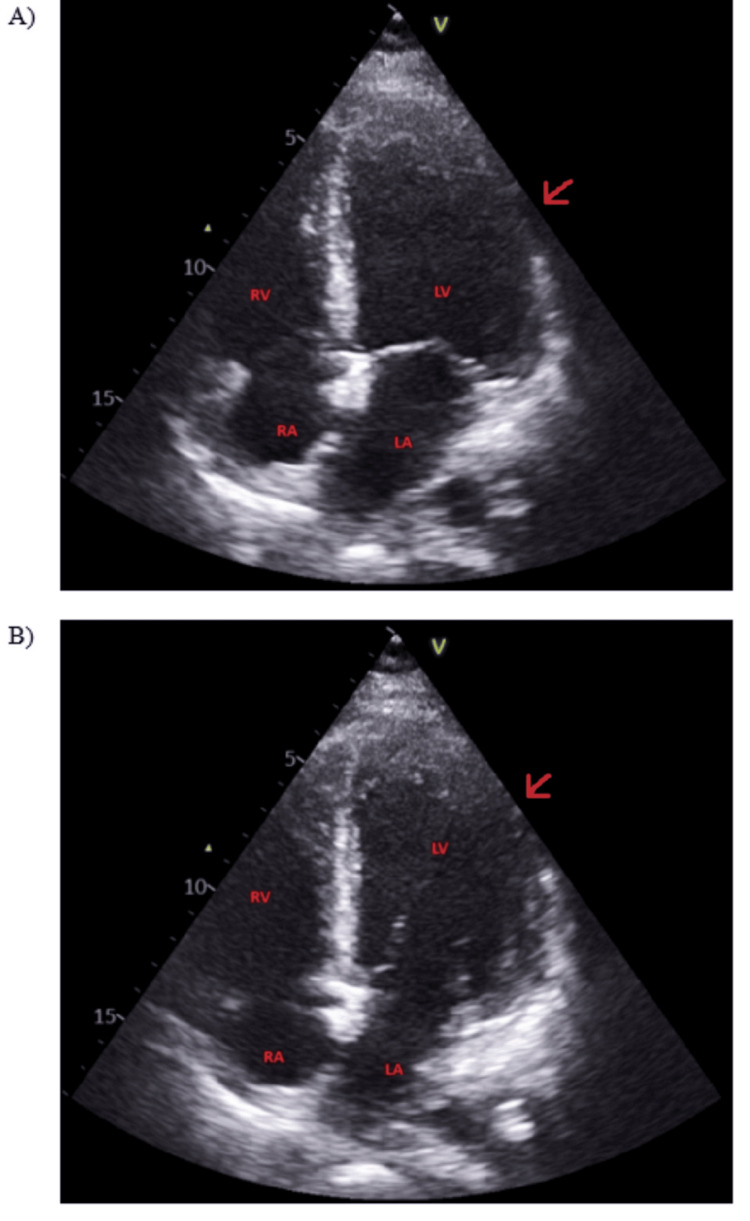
Transthoracic echocardiography performed shortly after ICU admission. The apical four-chamber view in (A) systole and (B) diastole The arrow in (A) shows severe global LV hypokinesia with no LV hypertrophy. The arrow in (B) comparing the LV size in diastole shows a severely impaired LV estimated ejection fraction of 20%-25% (visually estimated on a formal transthoracic echocardiography). In the same study, LV internal diameter in end-diastole was 52 mm, LV internal diameter in end-systole 44 mm, and LV fractional shortening was 15% ICU: intensive care unit; LV: left ventricular; RV: right ventricular; LA: left auricular; RA: right auricular
